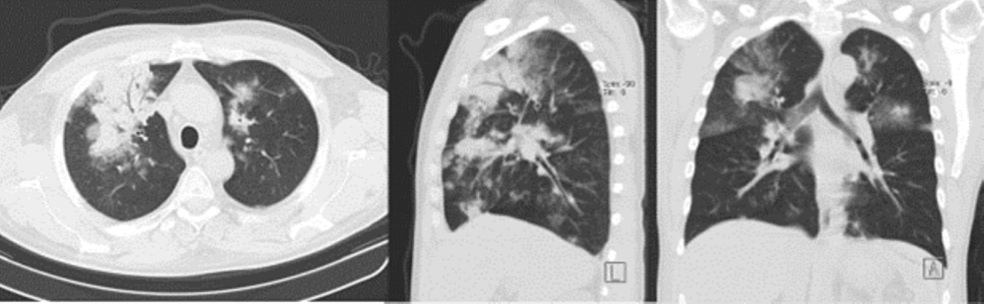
Chest CT scan Diffuse ground glass opacities and areas of consolidation with an air bronchogram in the medium right lobule and lower left lobule
